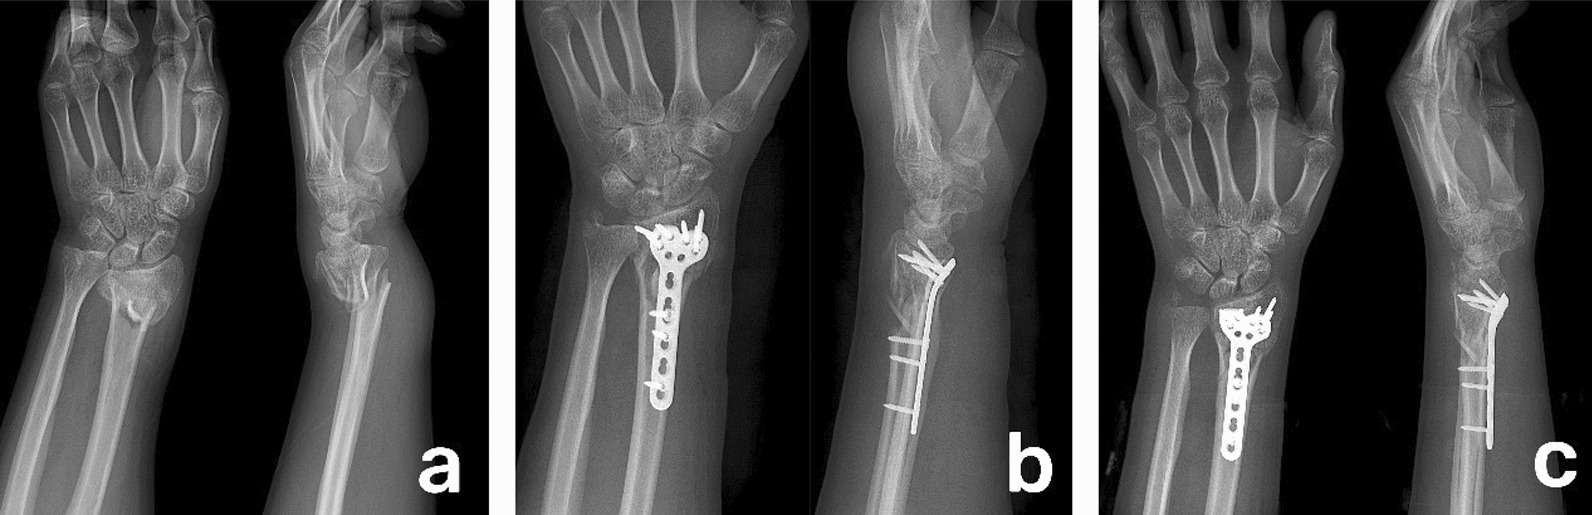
a Preoperative anterolateral X-ray of a patient with a C3 type comminuted fracture of the distal radius. b Postoperative first-day anterolateral X-ray with adequate reduction and fixation with a volar locking plate. c Anterolateral X-ray at 6 weeks postoperatively
